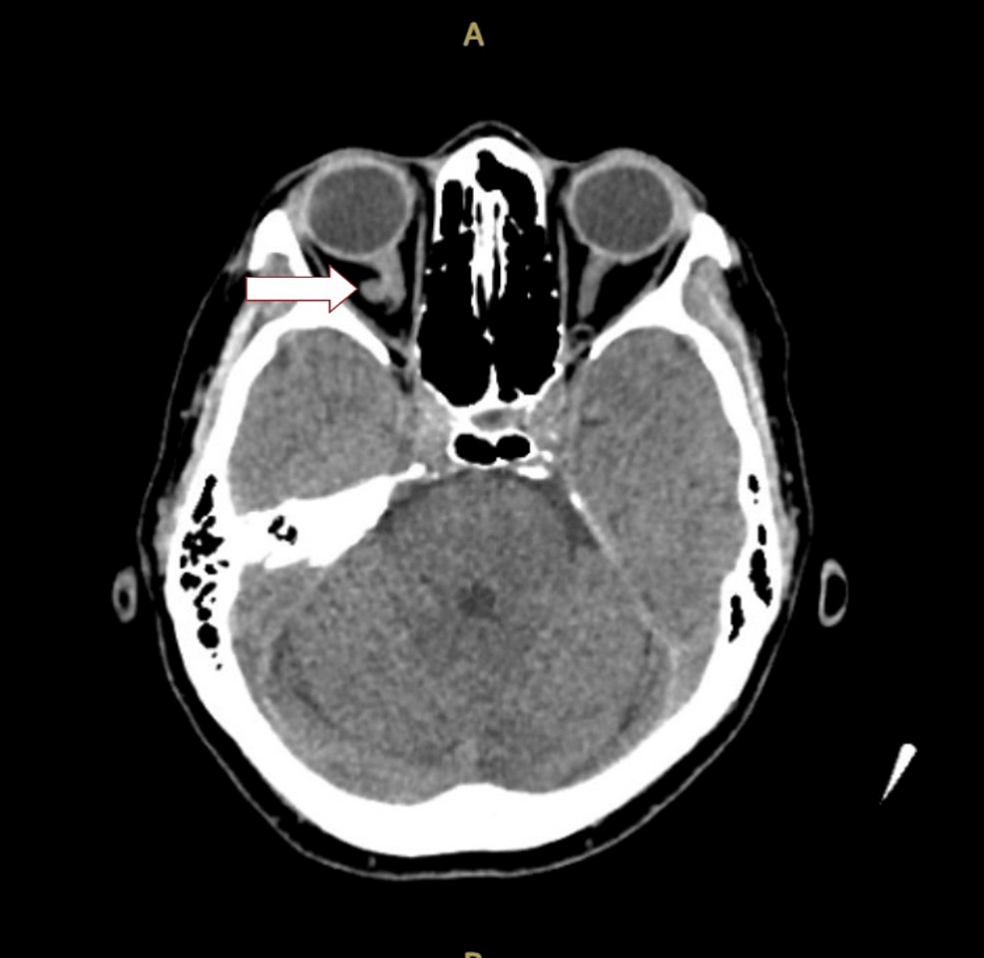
Plain CT brain shows a pedunculated soft tissue lesion at the lateral aspect of right optic nerve measuring 0.5 x 0.5 x 0.5 cm (AP x W X CC)
